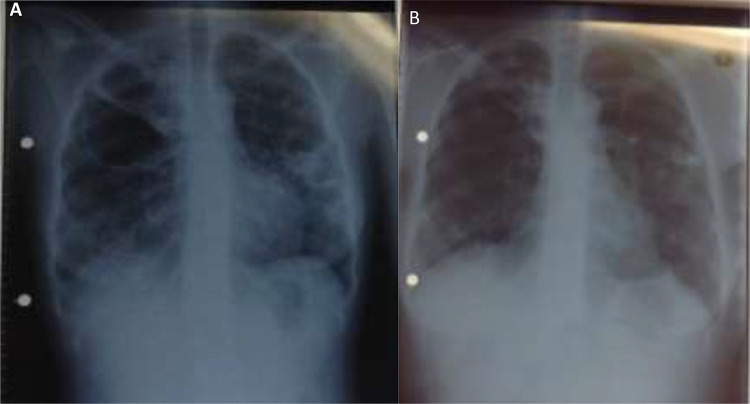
Plain film chest radiographs for patient three - Postero-Anterior view: (A) July 2020, showing right upper, mid-, and lower zone and left lower lobe consolidation and cavities in right upper and left lower lobes and right hilar lymphadenopathy. (B) January 2021, showing bilateral lower lobe fibrosis with fibro-cavitary changes of the right upper lobe.
